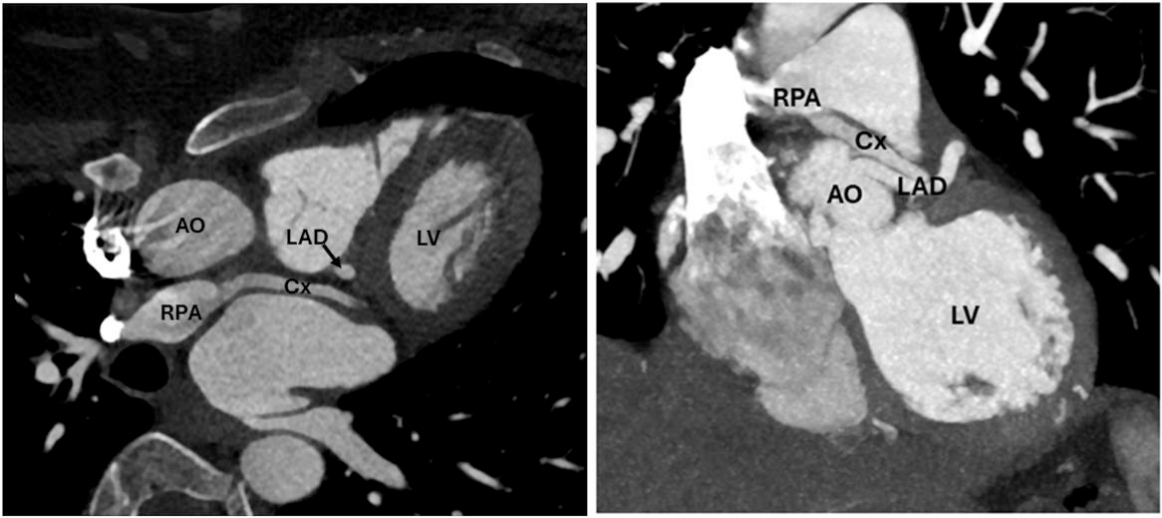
CT-scan showing anomalous arising of the circumflex coronary artery (cx) from the right pulmonary artery (RPA), while the left anterior descending artery (LAD) arises normally form the aorta (AO). LV, left ventricle.
